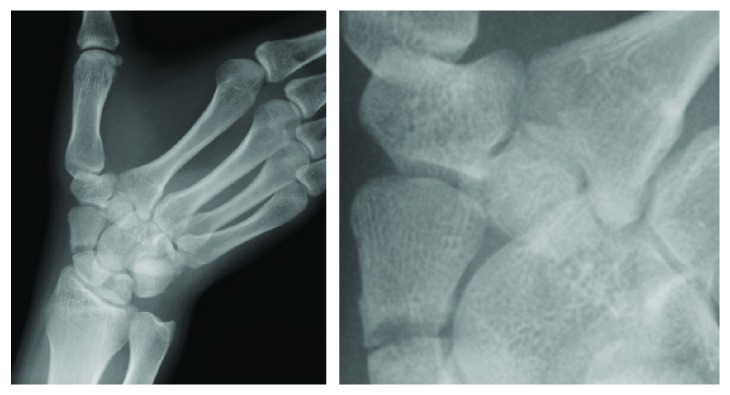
X-ray films showing displaced fracture of the trapezium and no other fractures.
